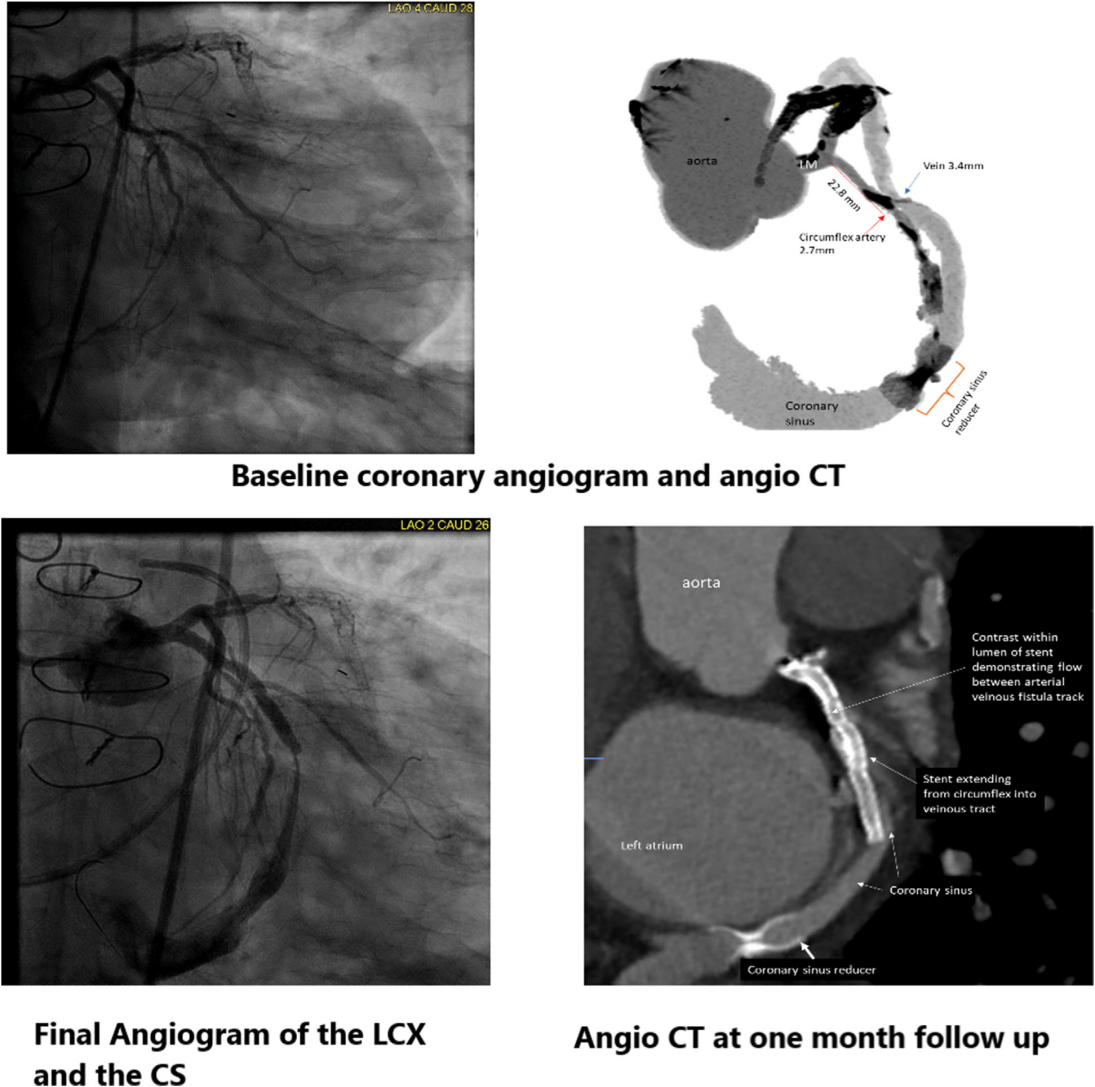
Baseline and Post Procedure Angio CT, and Angiograms (Top) Baseline coronary angiogram and coronary computed tomography angiography (angio CT) depicting the best point of communication to the coronary sinus (CS). (Bottom) Coronary angiogram after the establishment of the arteriovenous bypass and 1-month follow-up angio CT. LCX = left circumflex.
